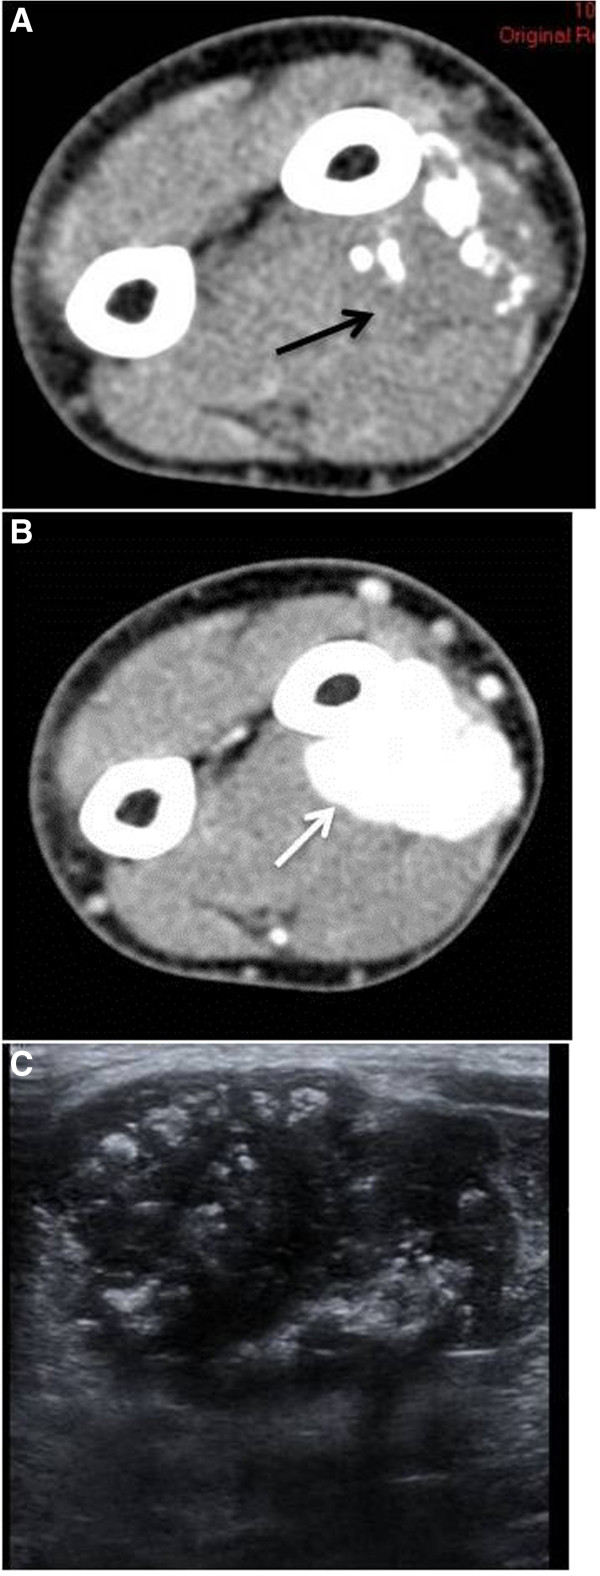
Unenhanced CT scan (A) displays a deep soft tissue mass with internal calcifications lying adjacent to the diaphysis of the left radius. The mass exhibits slightly lower attenuation compared to the neighboring muscles (black arrow). On contrast CT scan (B), the mass is homogeneously enhanced (white arrow). Ultrasound (C) demonstrates a hypoechoic mass containing multiple calcifications.
